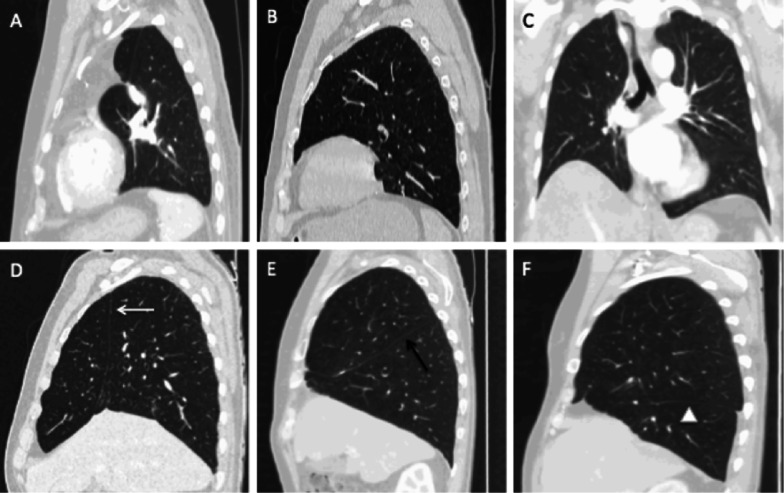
Scissure changes and normal displacement after lobectomy (parenchymal windowing). A: Sagittal CT image of a left upper lobectomy showing hyperinflated left lower lobe and mediastinal fat attraction without scissure. B: Sagittal CT image of a left lower lobectomy, note the absence of the oblique scissure. C: Coronal CT image after right lower and middle bilobectomy demonstrating elevated right hemidiaphragm and no visible scissure. D: Sagittal CT image of a right upper lobectomy with frontally oriented cranial neoscissure (white arrow). E Sagittal CT image after right middle lobectomy with oblique caudal neoscissure (black arrow). F: Sagittal CT image after right lower lobectomy with axially oriented posterior neoscissure (white arrowhead)C.
